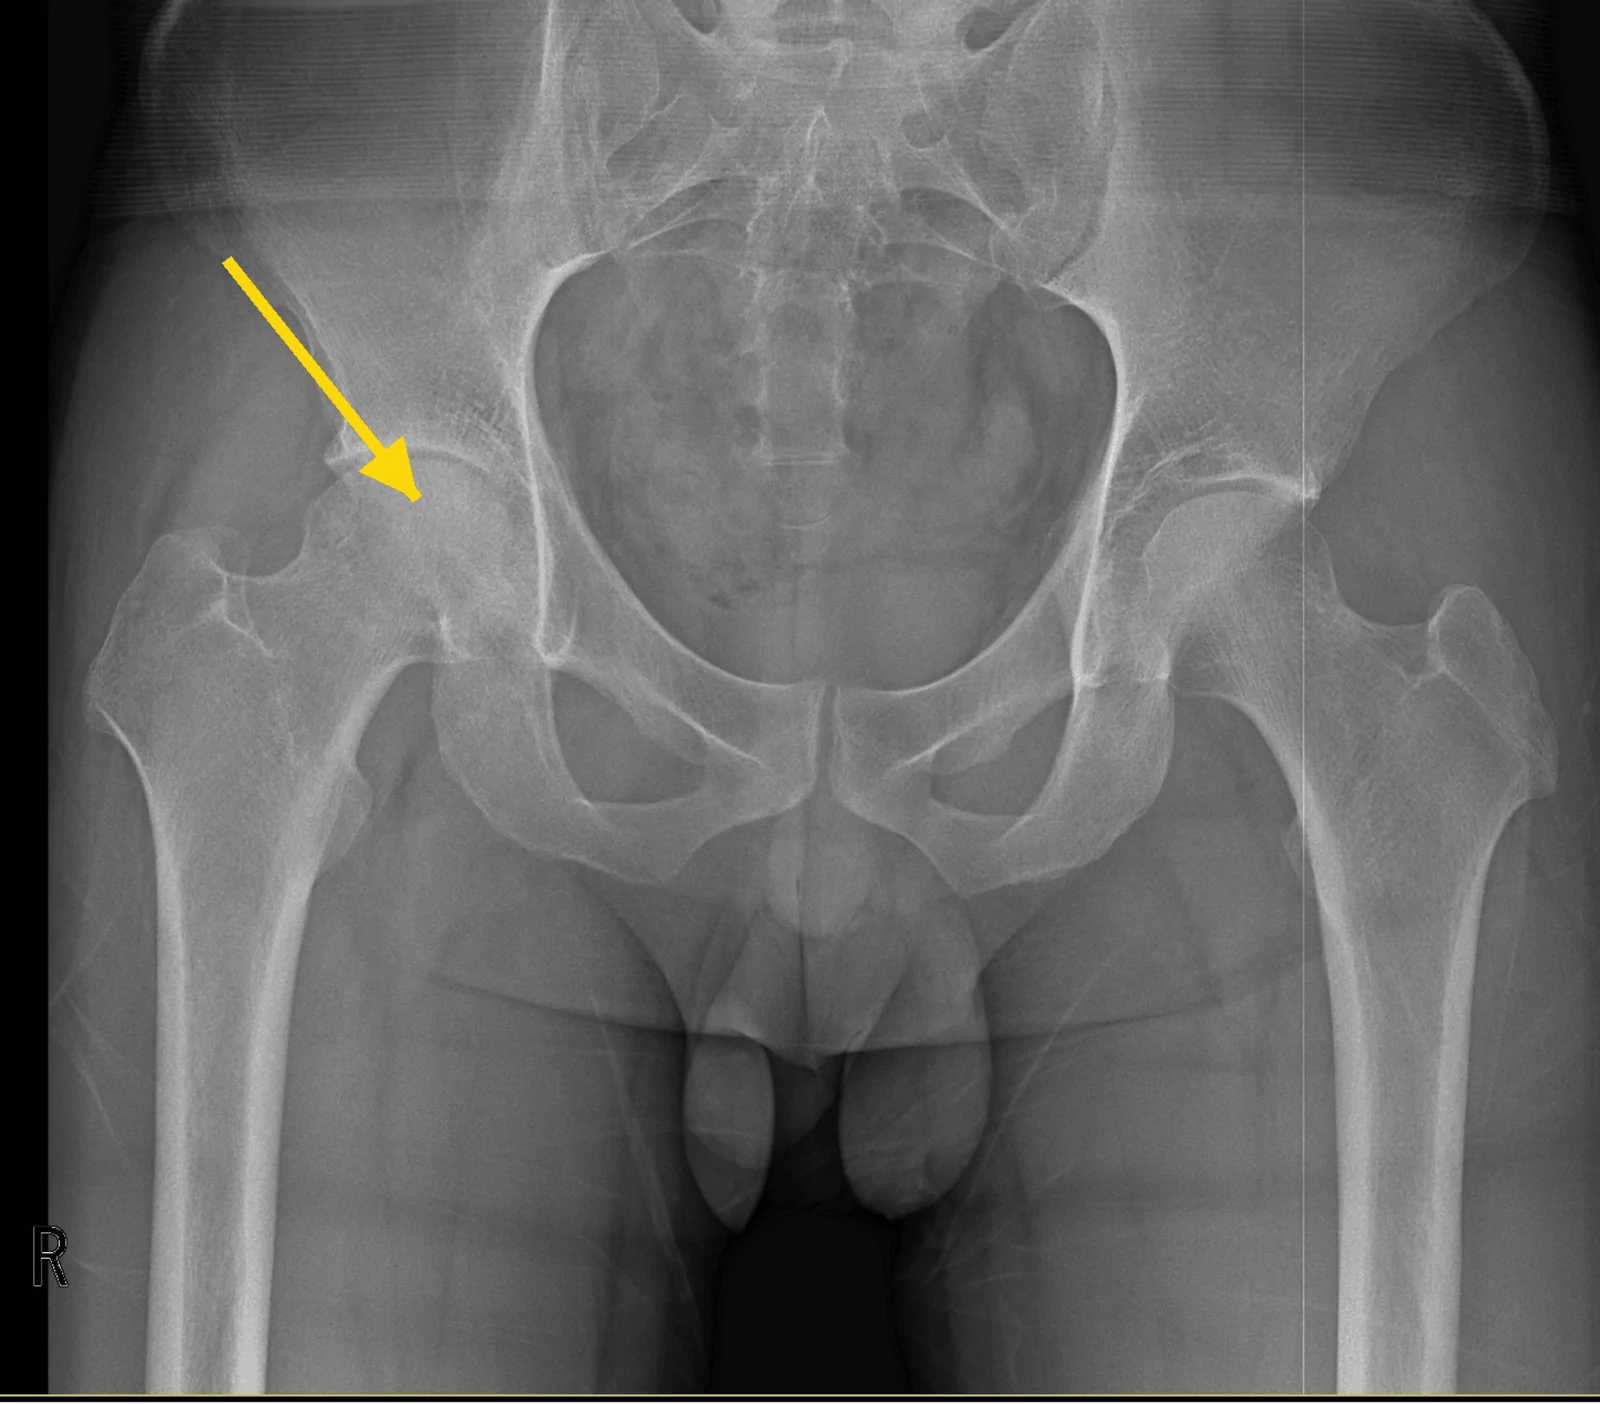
Anteroposterior pelvic radiograph at presentation The arrow indicates the superior articular surface of the right femoral head, showing mild osteoarthritic change and features suspicious for osteonecrosis.
